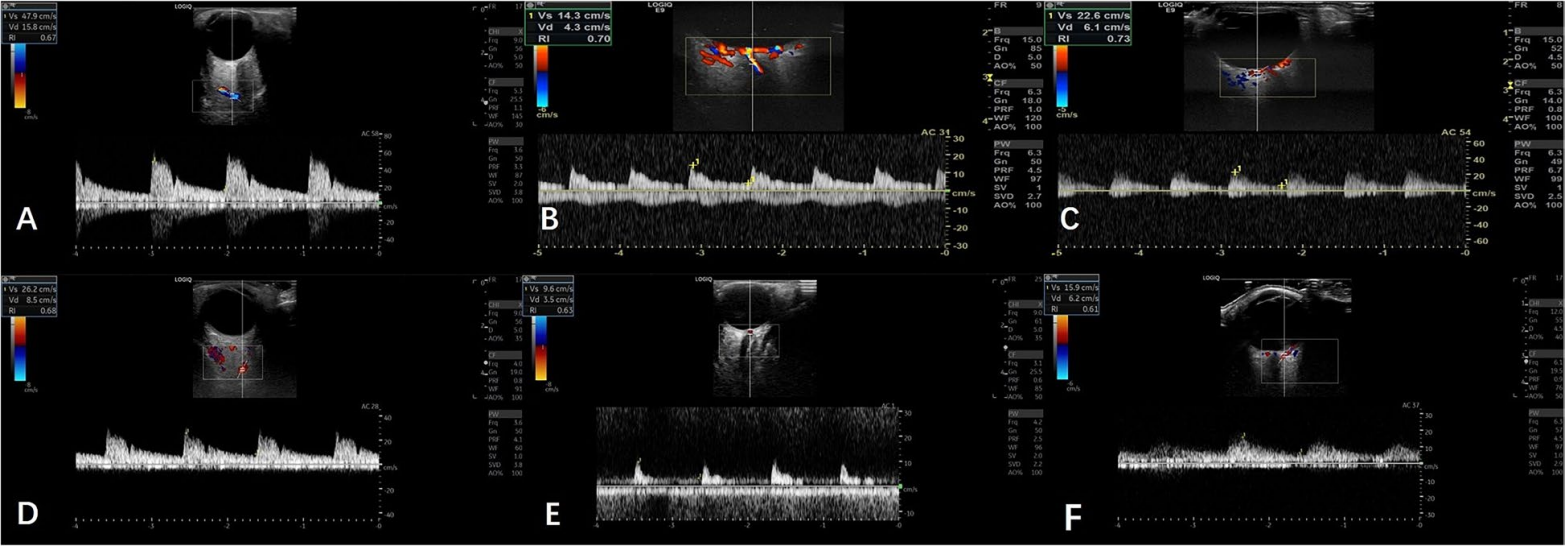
Color doppler ultrasound images of A ophthalmic artery, B central retinal artery, C posterior ciliary artery in active eyes. Color doppler ultrasound images of D ophthalmic artery, E central retinal artery, F posterior ciliary artery in non-active eyes
